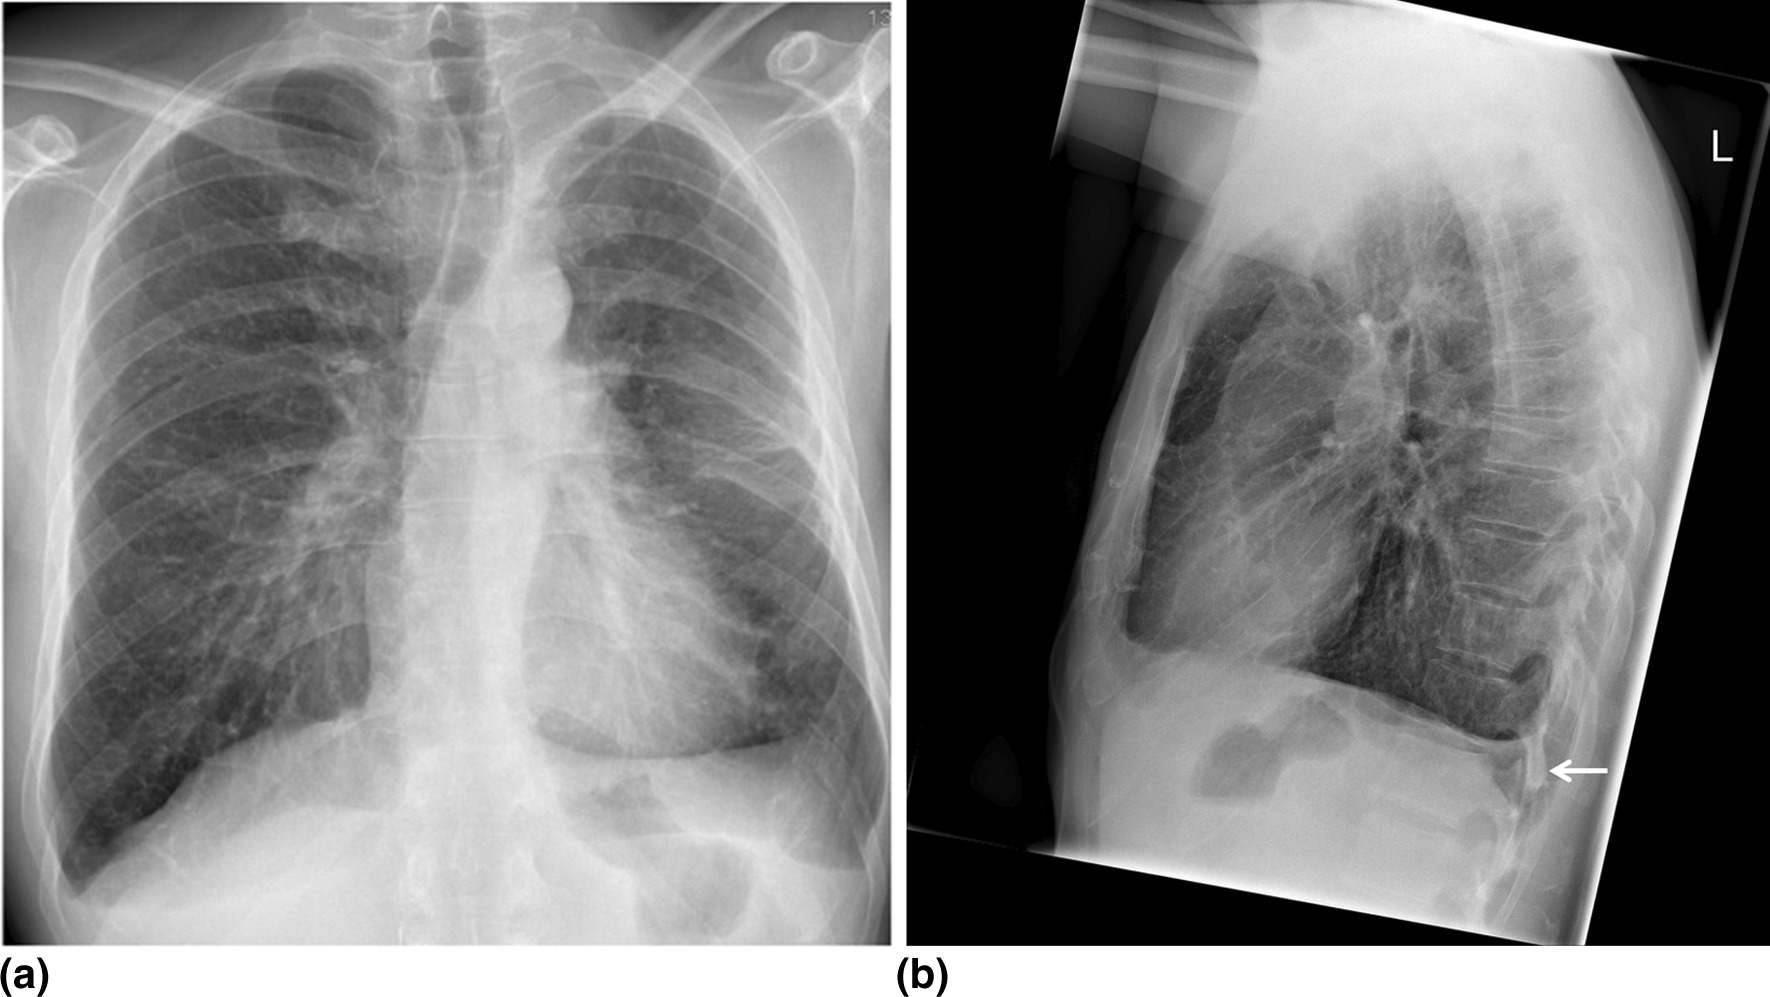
p.a. (a) and lateral chest X-ray (b) with an adhered costodiaphragmatic sinus (white arrow). Pleural thickening with fibrosis strands is seen on the left middle field
